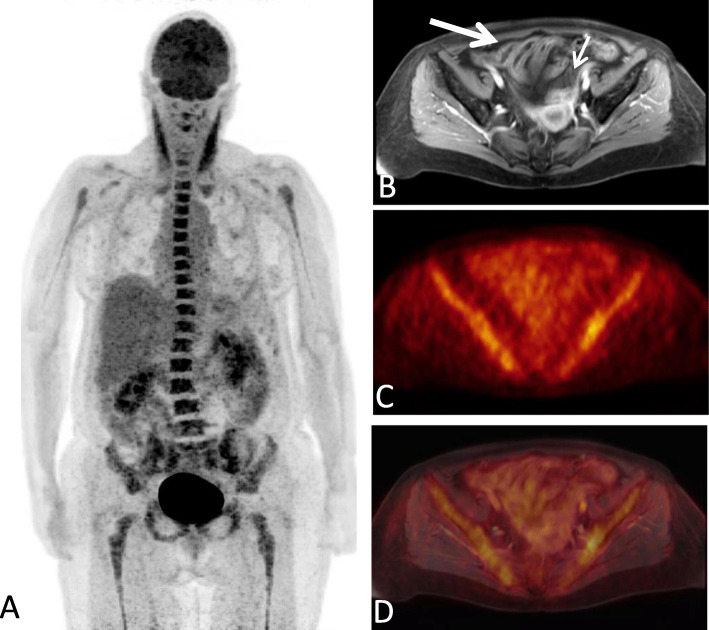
Whole-body MIP (A), contrast enhanced T1 weighted axial MRI sections (B), corresponding PET (C) and fused images (D) of a patient with acute intestinal GvHD (grade II). MR imaging shows contrast enhancement of ileal loops (thick arrow), mural stratification and hypervascular appearance of the mesentery (comb sign) (thin arrow) strongly suggesting presence of acute intestinal GvHD. ^18^F-FDG-PET, however, does not show increased uptake in the affected bowel segments (A,C,D)
